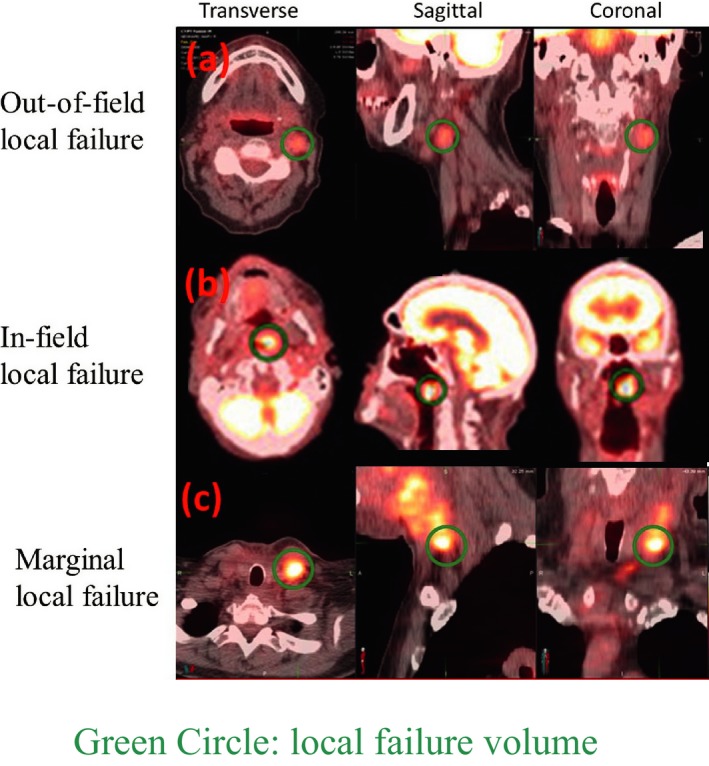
PET/CT scans showing out‐of‐field (*top panel*), in‐field (*middle panel*), and marginal local failure (*bottom panel*) in the transverse (*left column*), sagittal (*middle column*), and coronal (*right column*) plane. The dark green circles indicate the locations of local failure.
